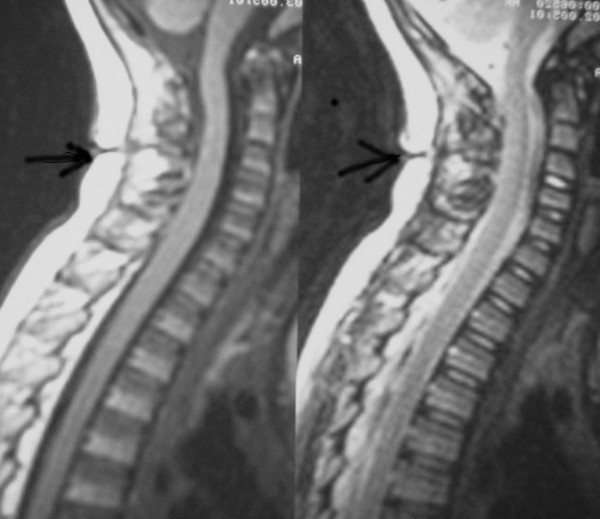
Sagittal T1-weighted MR image showing the opening of the dermal sinus at the level of C3–C4 and the extension of the tract outside the spinal canal.
